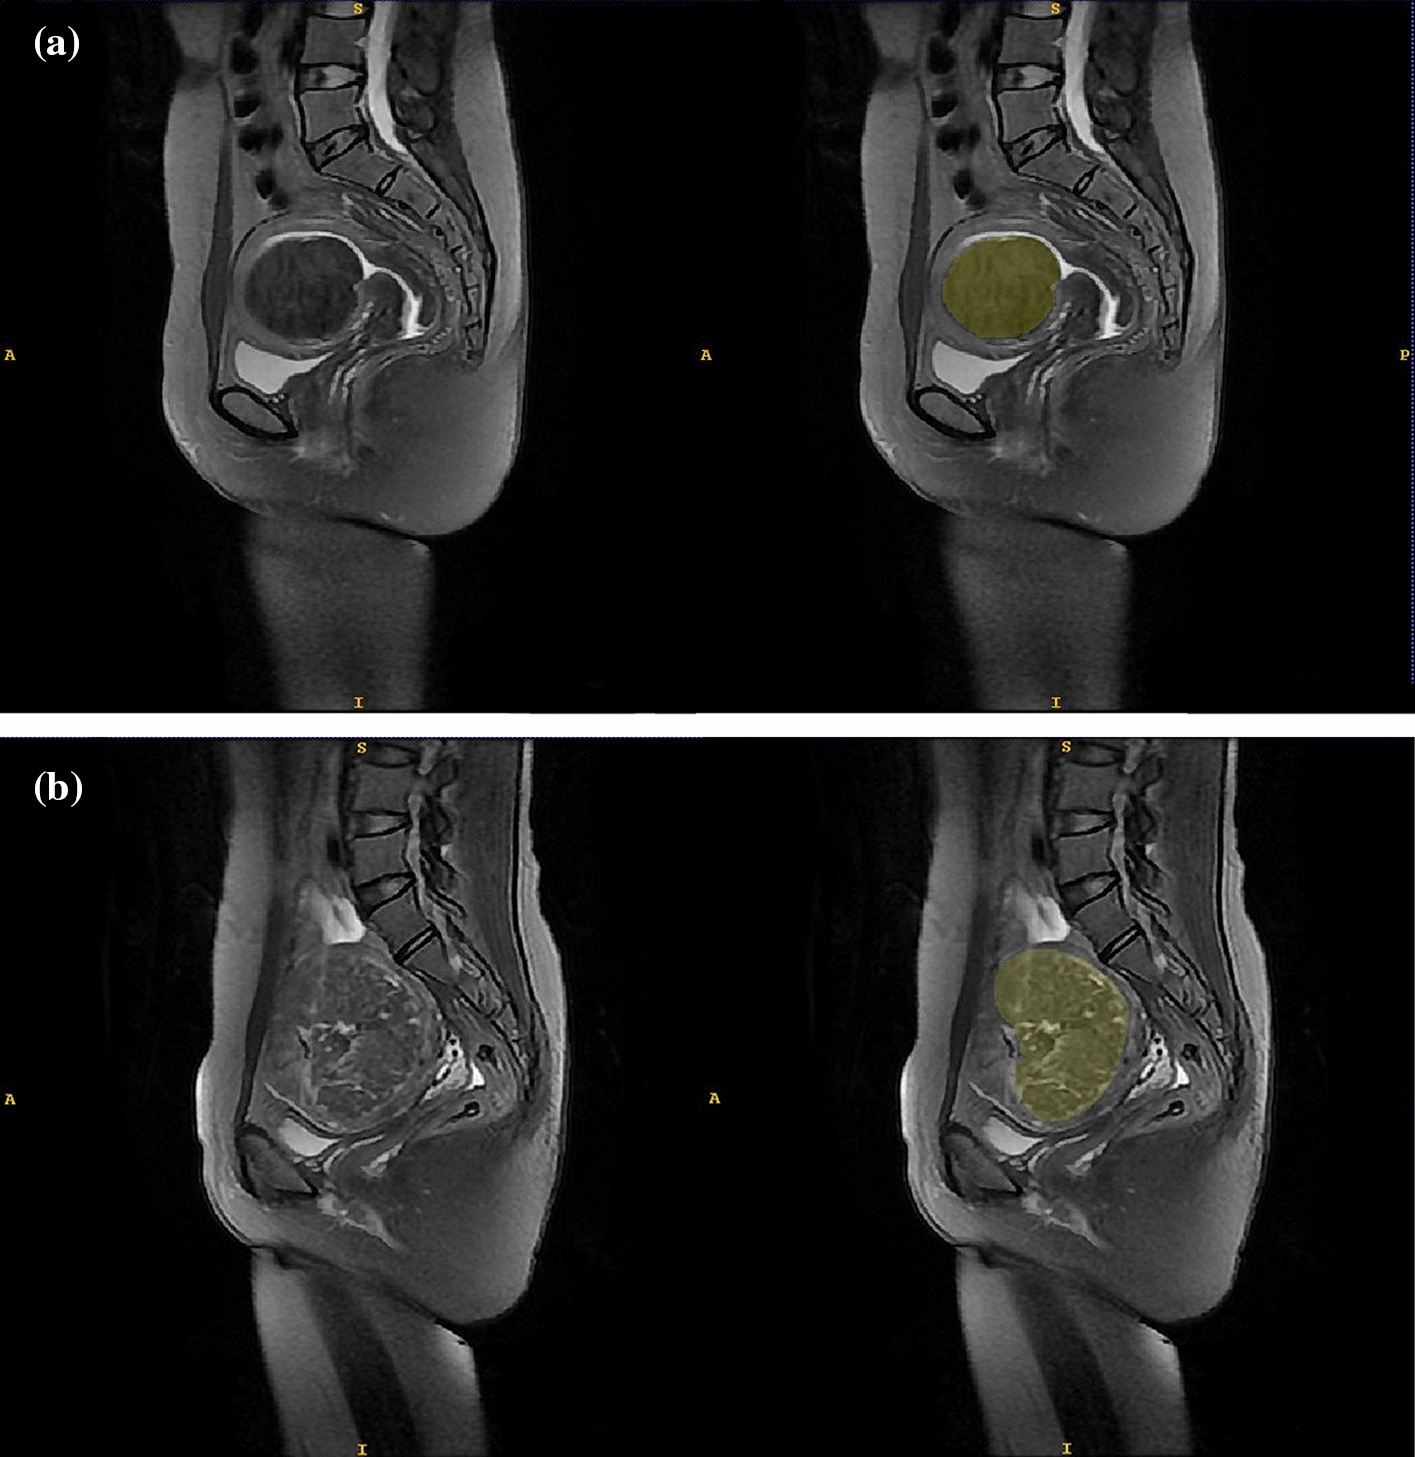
Example of T2-weighted magnetic resonance images (left) and tumor segmentation (right) of uterine fibroids obtained before treatment. a Hypo-intense fibroid; b heterogeneous hyper-intense fibroid
